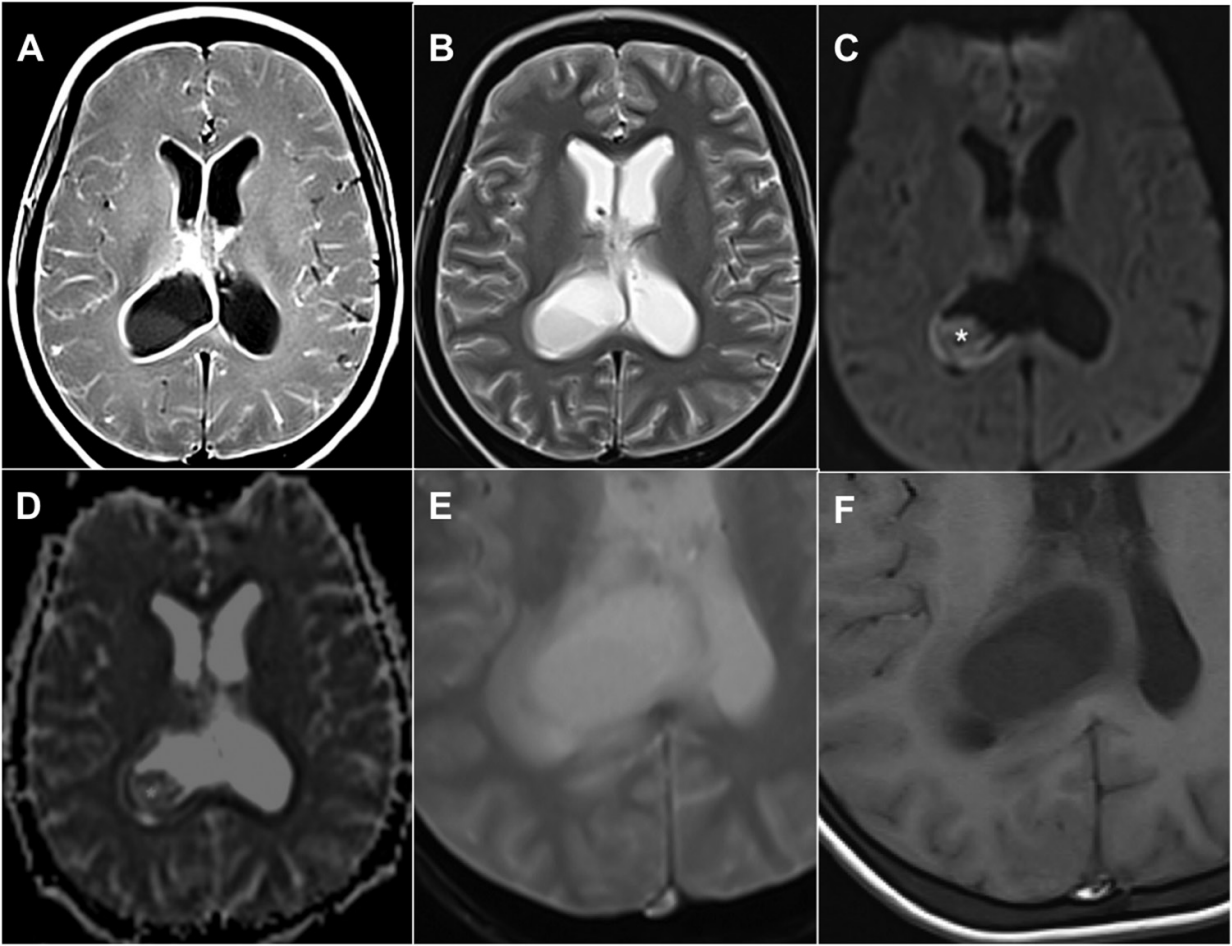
Axial magnetic resonance imaging of the brain shows hydrocephalus and the tuberculous abscess, which is a collection within the posterior horn of the right lateral ventricle. The (A) T1W post contrast image shows rim enhancement of the wall of the lesion, and the ependymal lining of both lateral ventricles and along the cortical gyri. (B) A T2W image shows hyperintense signal of the core of the lesion with an incomplete hypointense rim of the wall. (C) Diffusion-weighted image and the corresponding (D) apparent diffusion coefficient map demonstrate mild diffusion restriction (*) at the inferior aspect of the lesion. Close-up of axial images on (E) T2*-weighted gradient-echo and (F) T1W sequences. No evidence of a hypointense rim is seen in the gradient-echo image. T1W, T1-weighted; T2W, T2-weighted.
